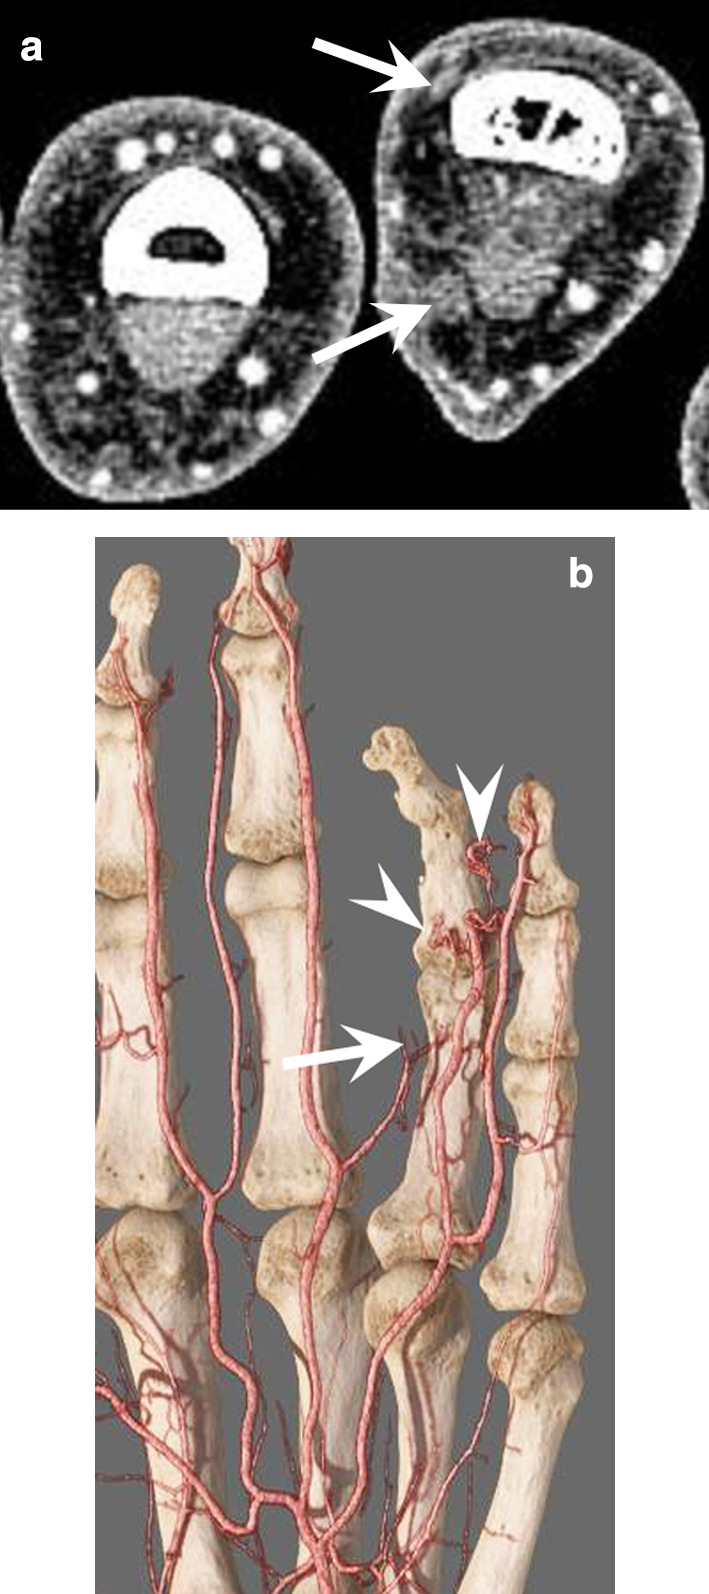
Traumatic occlusion of the radial digital arteries of the fourth finger in a 29-year-old man. a SHR-CTA at the level of the first phalanx showing the occlusion of the radiopalmar and radiodorsal digital arteries of the fourth finger (arrows) as well as a reduction of the venous flow. b GI showing the occlusion of the radiopalmar artery at the level of the first phalanx and of the ulnopalmar artery associated with corkscrew collaterals (arrowheads)
